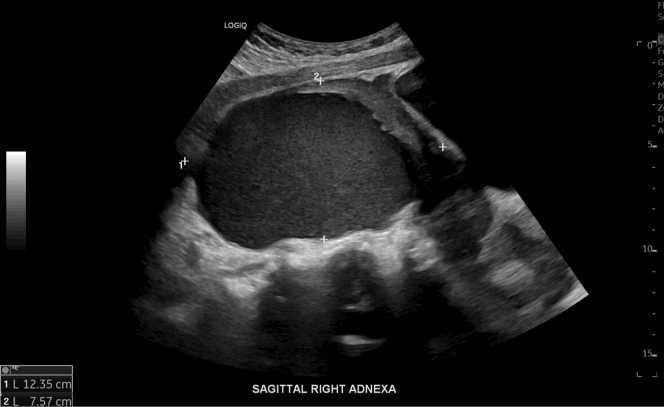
Transabdominal pelvic ultrasound demonstrating a large, complex hypoechoic mass in the right adnexa.
